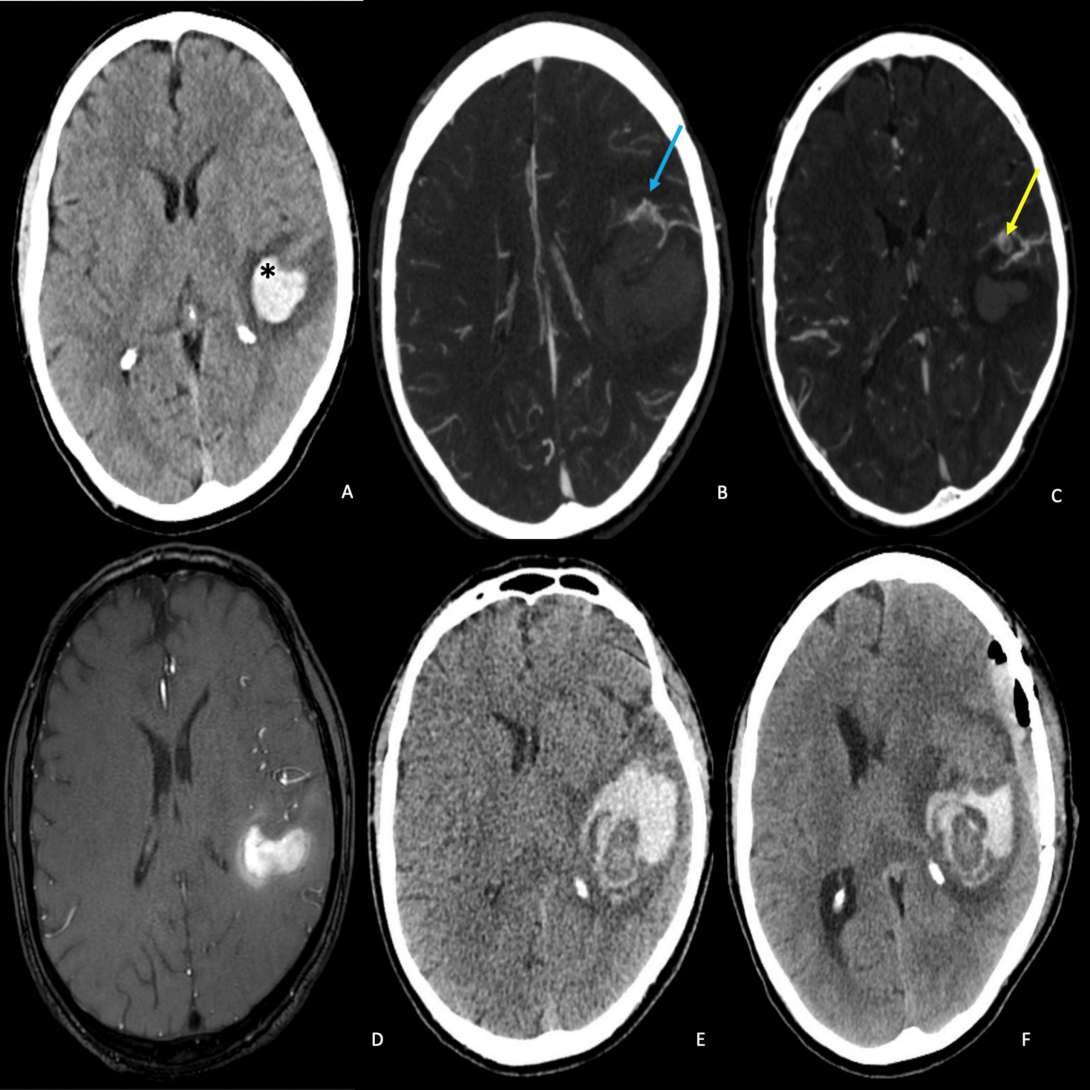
Serial imaging studies showing the disease progression. (A) CT brain (axial view) showed a hematoma (*) in the left parietal region near the Sylvian fissure. (B, C) Brain CTA revealed a nidus-like lesion (Blue arrow), a rounded structure (Yellow arrow) with prominent cortical vessels in this region, suggestive of an arteriovenous malformation (AVM). (D) Gadolinium-enhanced brain MRI (axial view) showed evolving methemoglobin with mild perifocal edematous changes, consistent with evolving methemoglobin. (E) Brain CT was repeated on day 17 after the initial bleed showing a recurrent bleed. (F) Further rebleeding in the surgical cavity was noted, along with postoperative changes, including a mild subdural residual hematoma and pneumocephalus.
